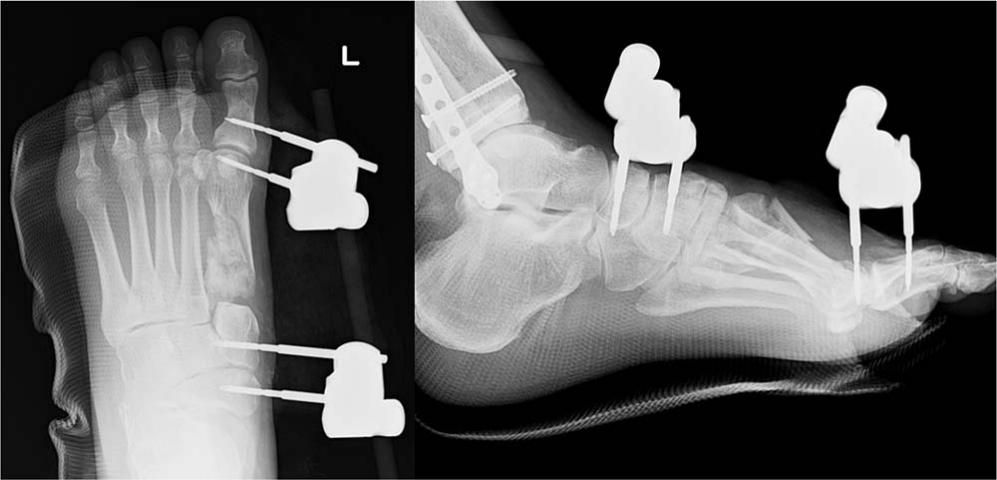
Radiography images immediately after the first surgery.
